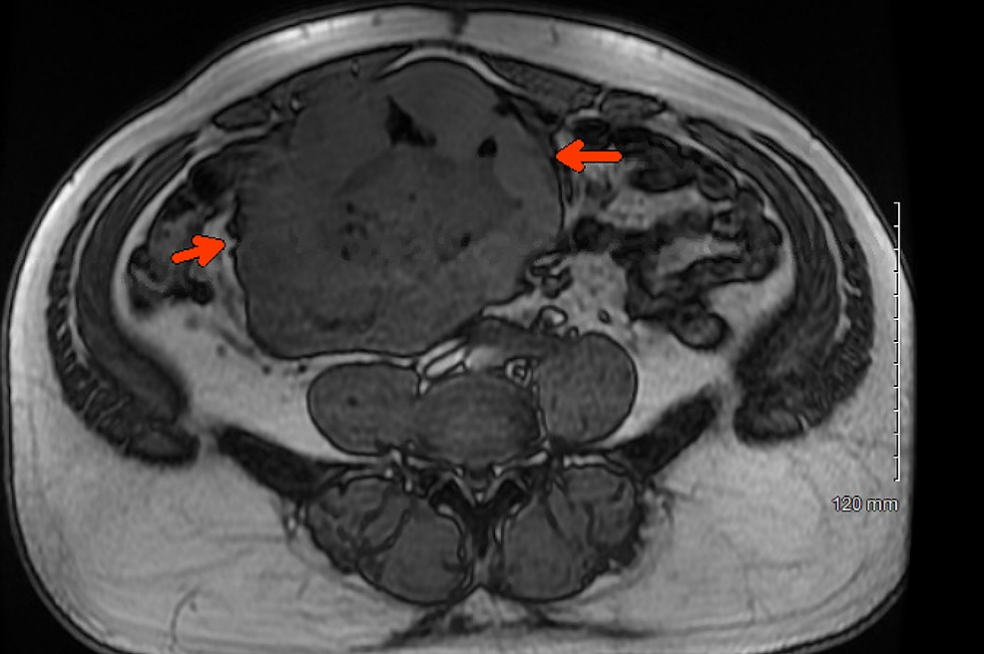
Magnetic resonance imaging of the abdomen showing a large necrotic enhancing mass (arrows) centered in the small bowel mesentery.
